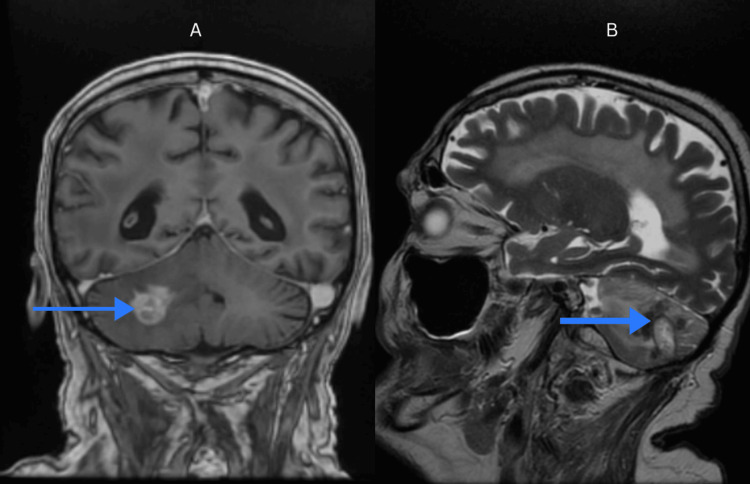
MRI sagittal scan. MRI brain demonstrating at the level of the right cerebellar hemisphere, a native hypodense area (blue arrow), with a heterogeneous appearance, with some hyperdense images and intracranial aerocele, with a compressive effect on V4.
